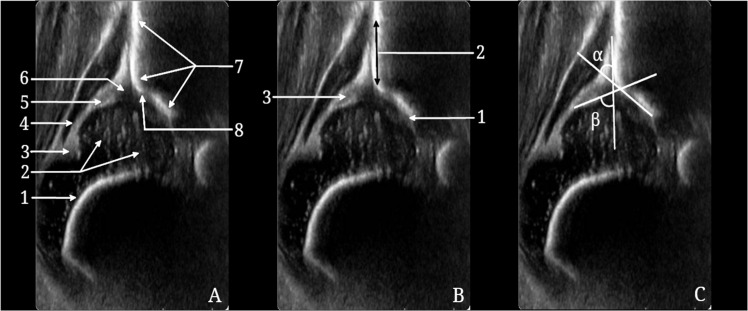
An ultrasound image of the hip showing Checklist 1, Checklist 2, and the measurement of angles in the Graf method. (A) Checklist 1 for anatomy: 1 - Chondro-osseous junction, 2 - Femoral head, 3 - Synovial fold, 4 - Joint capsule, 5 - Acetabular labrum, 6 - Hyaline cartilage roof, 7 - Bony acetabular roof, 8 - Bony rim: turning point from concavity to convexity. (B) Checklist 2 for image plane: 1 - Lower limb of the ilium, 2 - Standard sectional plane, 3 - Acetabular labrum. (C) Alpha and beta angles.
